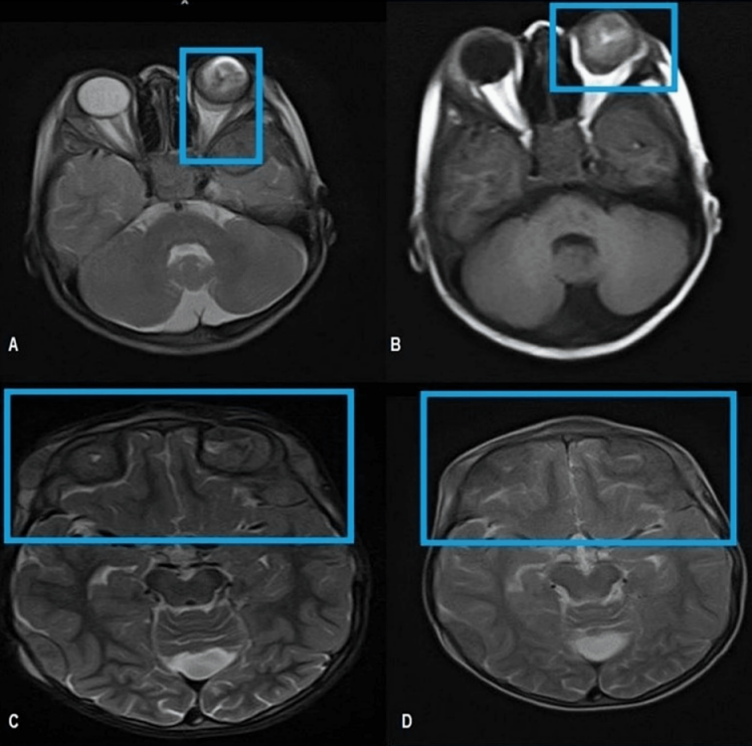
Enlarged left orbit showing intraocular retro globular mass with retinal detachment and T1W bright signal suggesting internal hemorrhage. A: T2W Axial MRI brain reveals an intraocular mass in the posterior aspect of the left globe abutting the retina returning heterogeneous hypointense signals. B: T1W axial MRI brain shows an intraocular lesion with a V-shaped T1W hyperintense signal in the posterior left globe reflecting hemorrhagic retinal detachment. Note the extraocular extension of the tumor through the sclera resulting in the deformed globe. C and D: Axial MRI T2W images cranial section reveals extra-axial low signal intensity masses in the bi-frontal region and left scalp.
